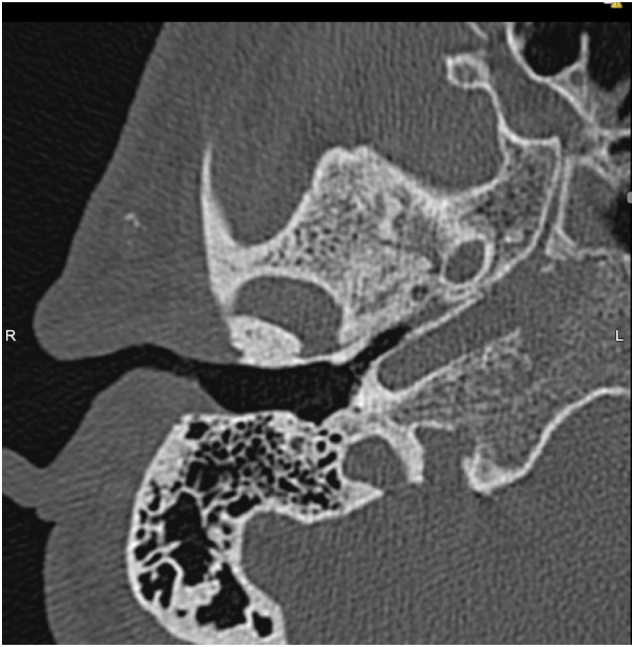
Fused PET/CT image showing disease progression regarding the extension of the active bone infection involving the left side of the skull base bones to currently involve the sphenoid body and right petrous with currently detected hypermetabolic nasopharyngeal mucosal thickening and hypermetabolic activity at prevertebral space and right carotid space.
